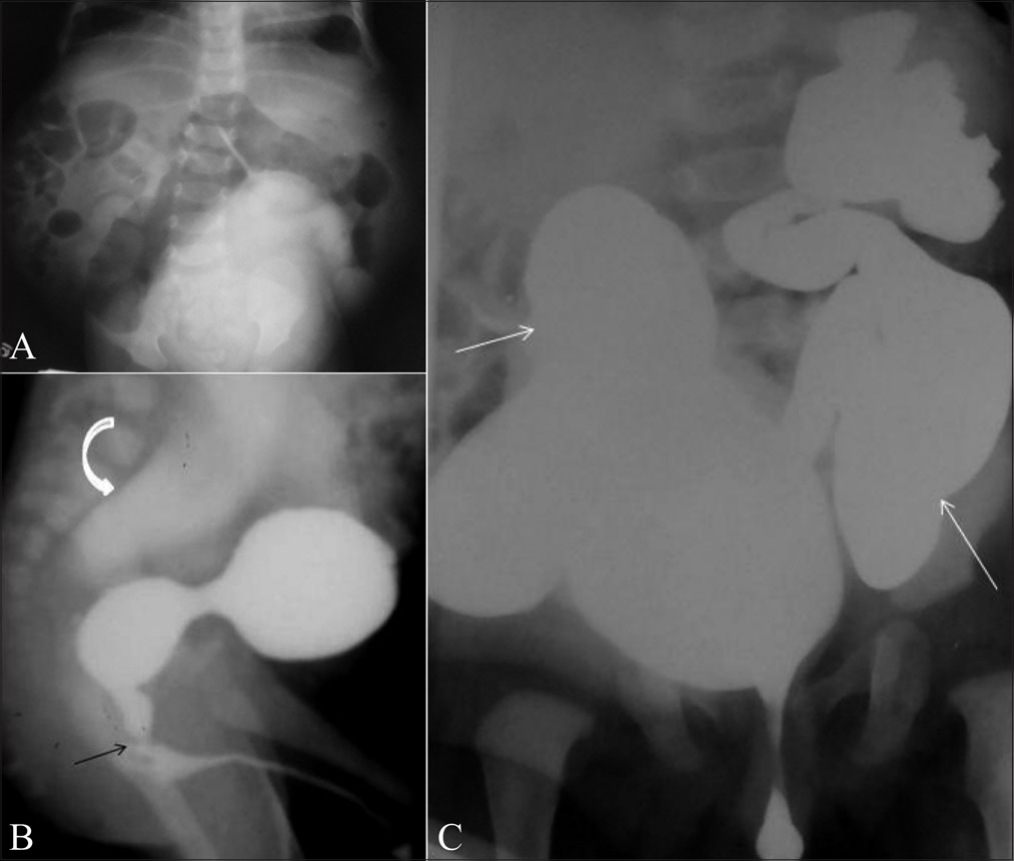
Prune belly syndrome in a newborn. The infant had a lax and winkled anterior abdominal wall and the scrotal sacs were empty. A frontal abdominal radiograph (delayed postcontrast image, A) reveals the lax and patulous abdominal wall manifested by the distended flanks. An oblique VCUG image (B) reveals an elongated, dilated, and patulous posterior urethra, with tapered transition into a normal anterior urethra (arrow). Note the grossly dilated ureters and the vesicoureteric reflux (curved arrow). It is the tapered transition of the posterior urethra that helps differentiate this condition from PUV, in which there is an abrupt transition. There is bilateral grade V vesicoureteric reflux (arrow) and grossly tortuous laterally placed ureters (C), in this frontal VCUG image
